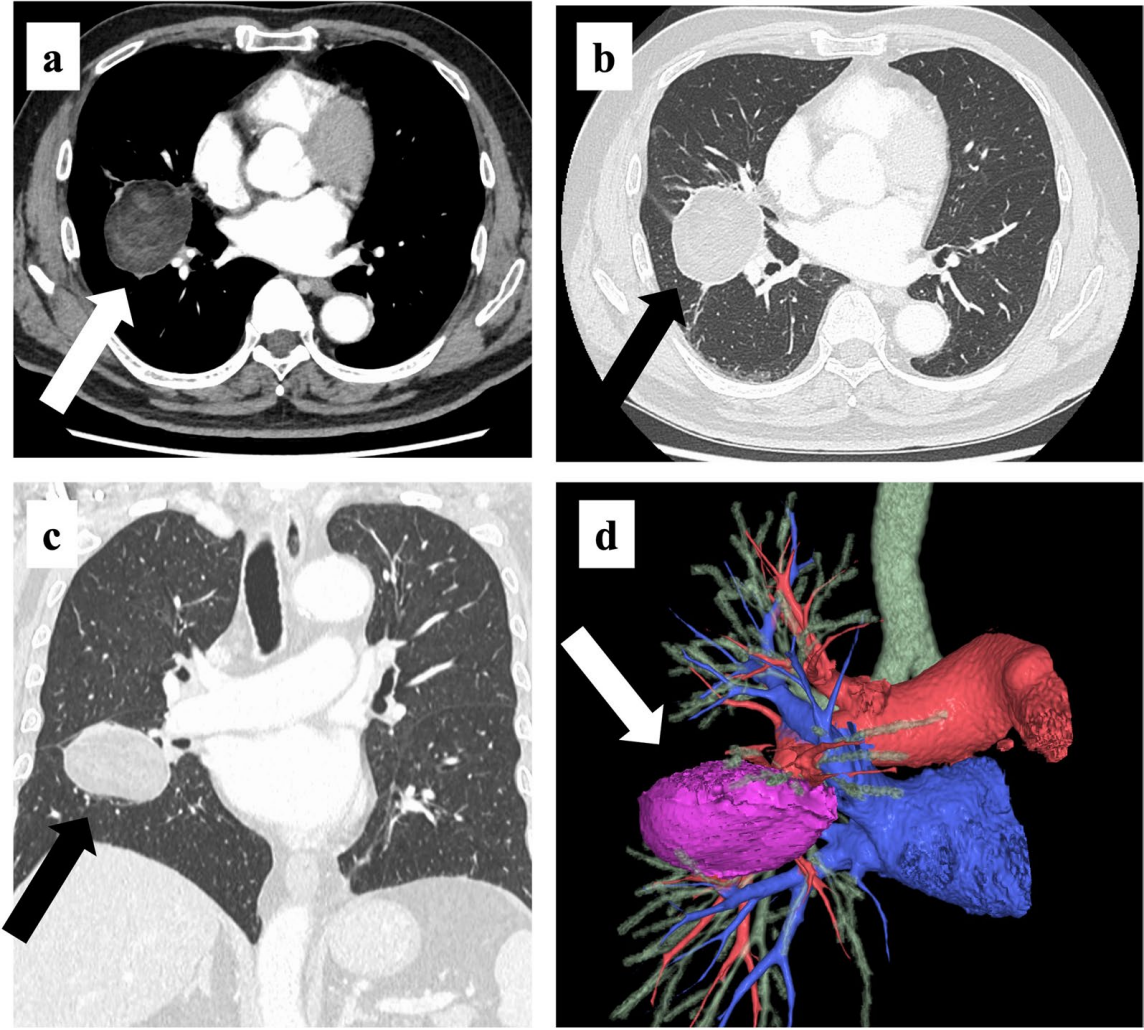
Chest computed tomography (CT) findings. a–c A 53 × 48-mm mass lesion with mixed fatty density can be seen spanning the middle and lower lobes of the right lung. d Three-dimensional (3D) reconstruction showing a mass lesion spanning the middle and lower lobes of the right lung with a normal bronchial branch
